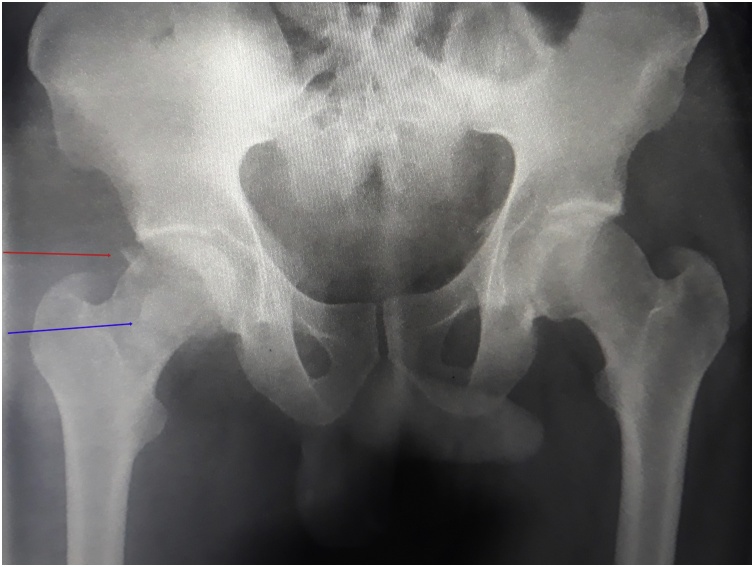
Immediate pelvic X-ray showing a non-reduction of right hip because of a non-reduced Pipkin II fragment (blue arrow) with a small superior fragment (red arrow).
